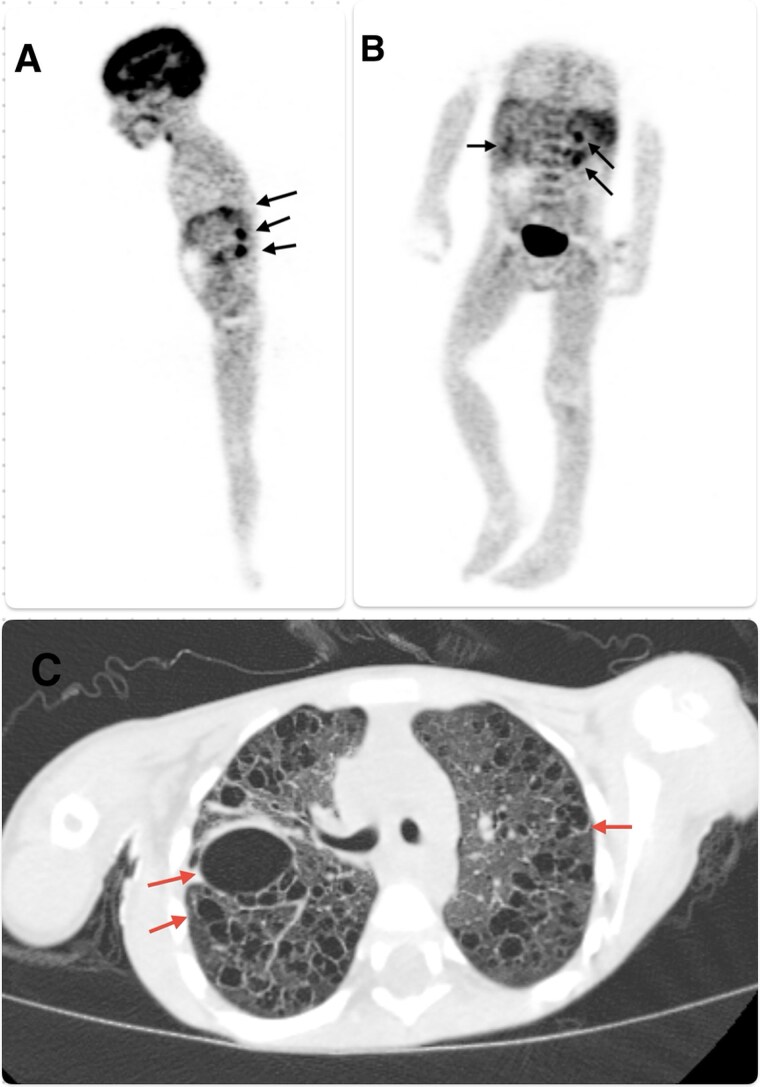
Whole-body FDG-PET/CT and axial chest CT in multisystem LCH. (A) Sagittal maximum-intensity-projection (MIP) FDG-PET image demonstrating mildly increased FDG uptake (black arrows) in extensive bilateral pulmonary cystic lesions. (B) Coronal MIP FDG-PET image showing FDG-avid pulmonary cysts (black arrows), mildly metabolically active subcentimetre to enlarged supra- and infradiaphragmatic lymphadenopathy, and hepatosplenomegaly with diffuse splenic hypermetabolism. (C) Axial high-resolution CT through the upper lobes depicting numerous thin-walled cysts of varying sizes in both lungs (red arrows), including a large right upper-lobe cyst (central arrow). Abbreviations: FDG, 2-deoxy-2-[^18F]fluoro-D-glucose; PET, positron-emission tomography; CT, computed tomography; MIP, maximum-intensity projection; LCH, Langerhans cell histiocytosis; HRCT, high-resolution CT.
